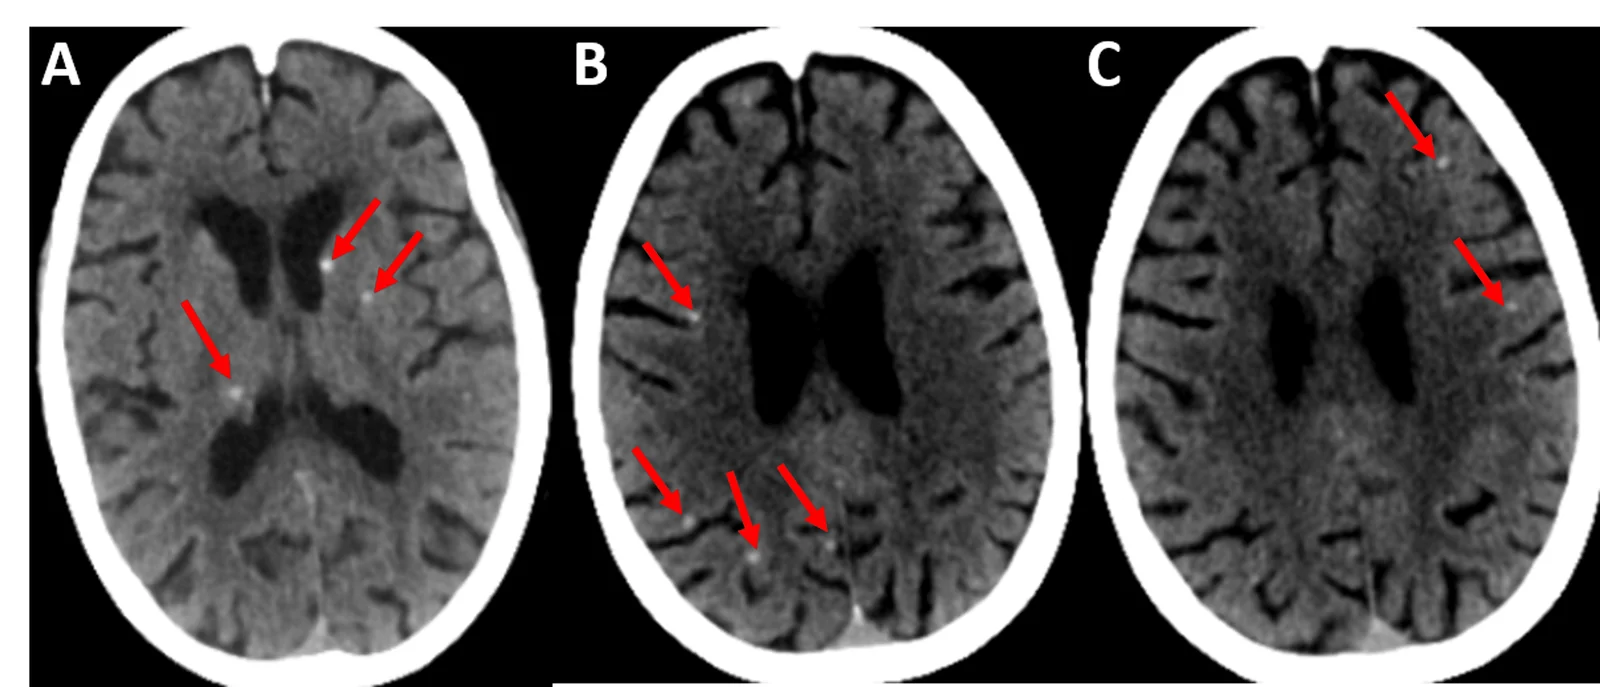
Axial non-contrast brain computed tomography scan showing multiple small hyperdense rounded lesions (A) Left caudate, lentiform nuclei, and right thalamus. (B) Right frontal and parietal cortex. (C) Left frontal cortex.
